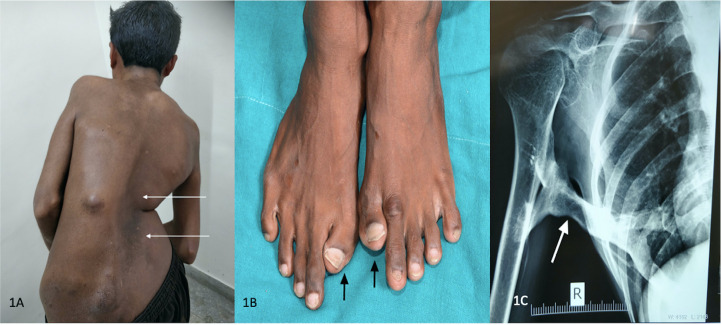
Clinical photograph showing kyphoscoliosis (A) and short first toe in both feet (B) and radiograph showing ossification of right latissimus dorsi muscle (C).
